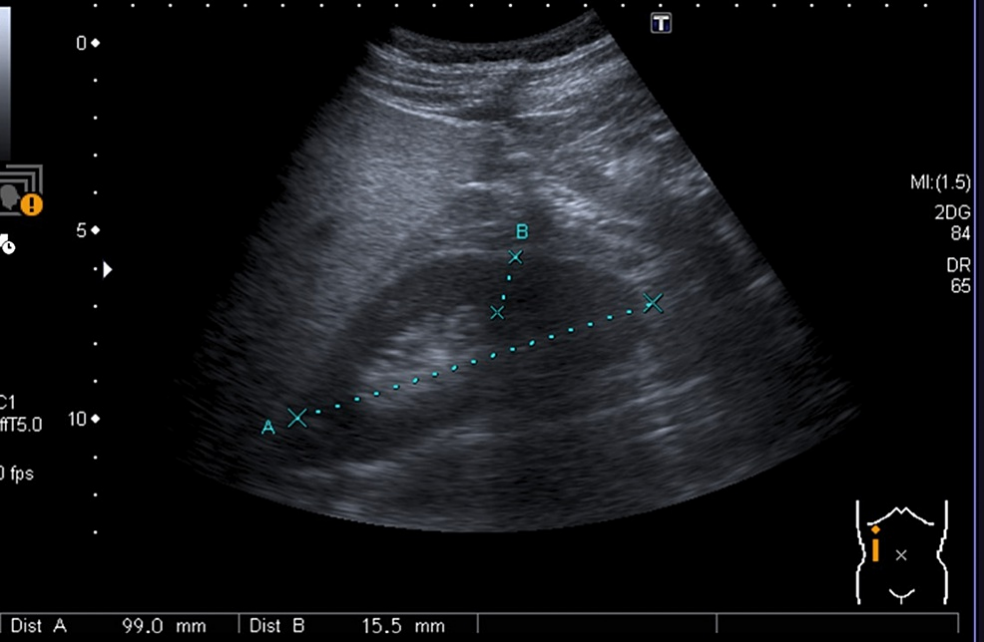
Abdominal ultrasound Kidney and right lobe of the liver with apparently standard dimensions.
